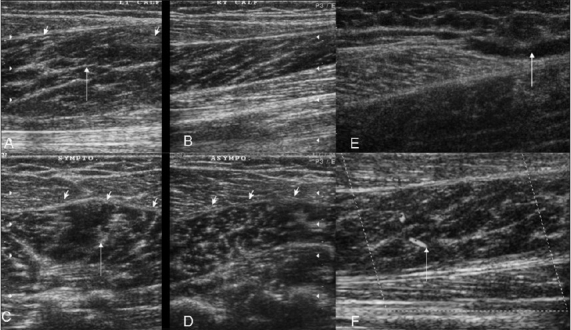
Case 6. Symptomatic (A) and asymptomatic (B) longitudinal and symptomatic (C) and asymptomatic (D) transverse images demonstrate swelling and loss of the normal pennate appearance (arrows) on the left side. Fluid is seen (arrow) near the musculotendinous region on a longitudinal extended field of view image (E). Increased vascularity is seen on the power Doppler (F)
